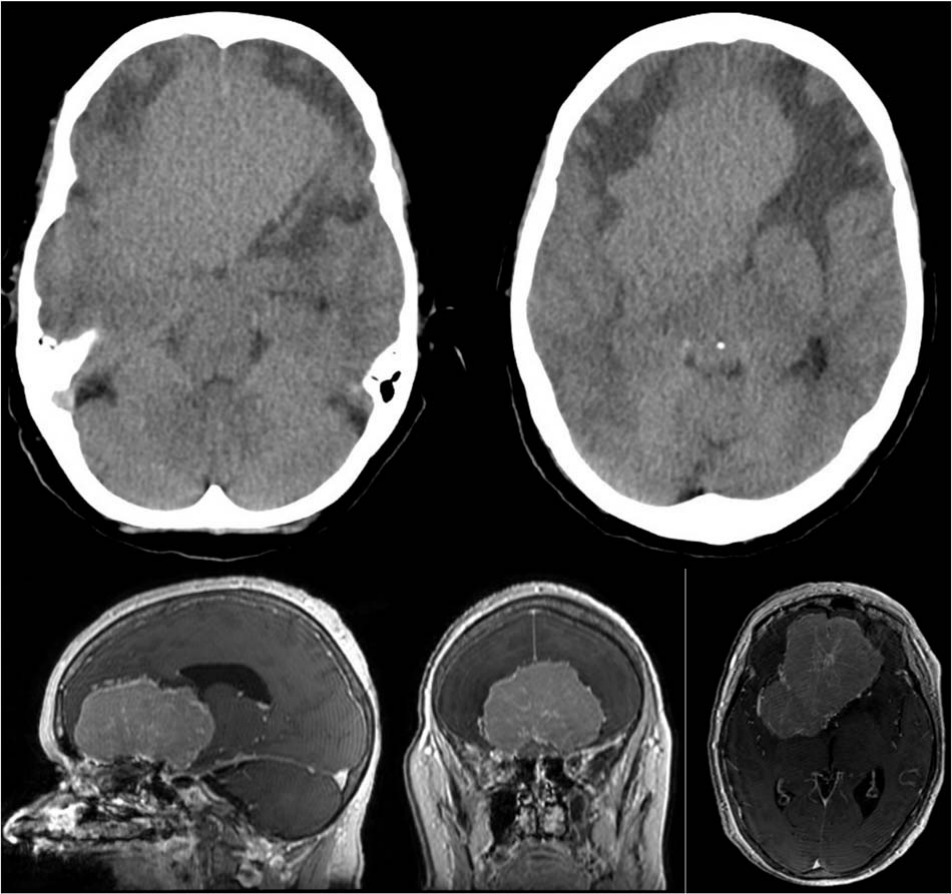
Showing the CT imaging with a hypodense fronto-basal lesion with finger-shaped perifocal edema and the T1-weighted MRI image with homogenous contrast-enhancing frontobasal lesion demonstrating mild compression on both lateral ventricles and posterior displacement of the right middle cerebral artery (MCA) without evidence of hydrocephalus.
